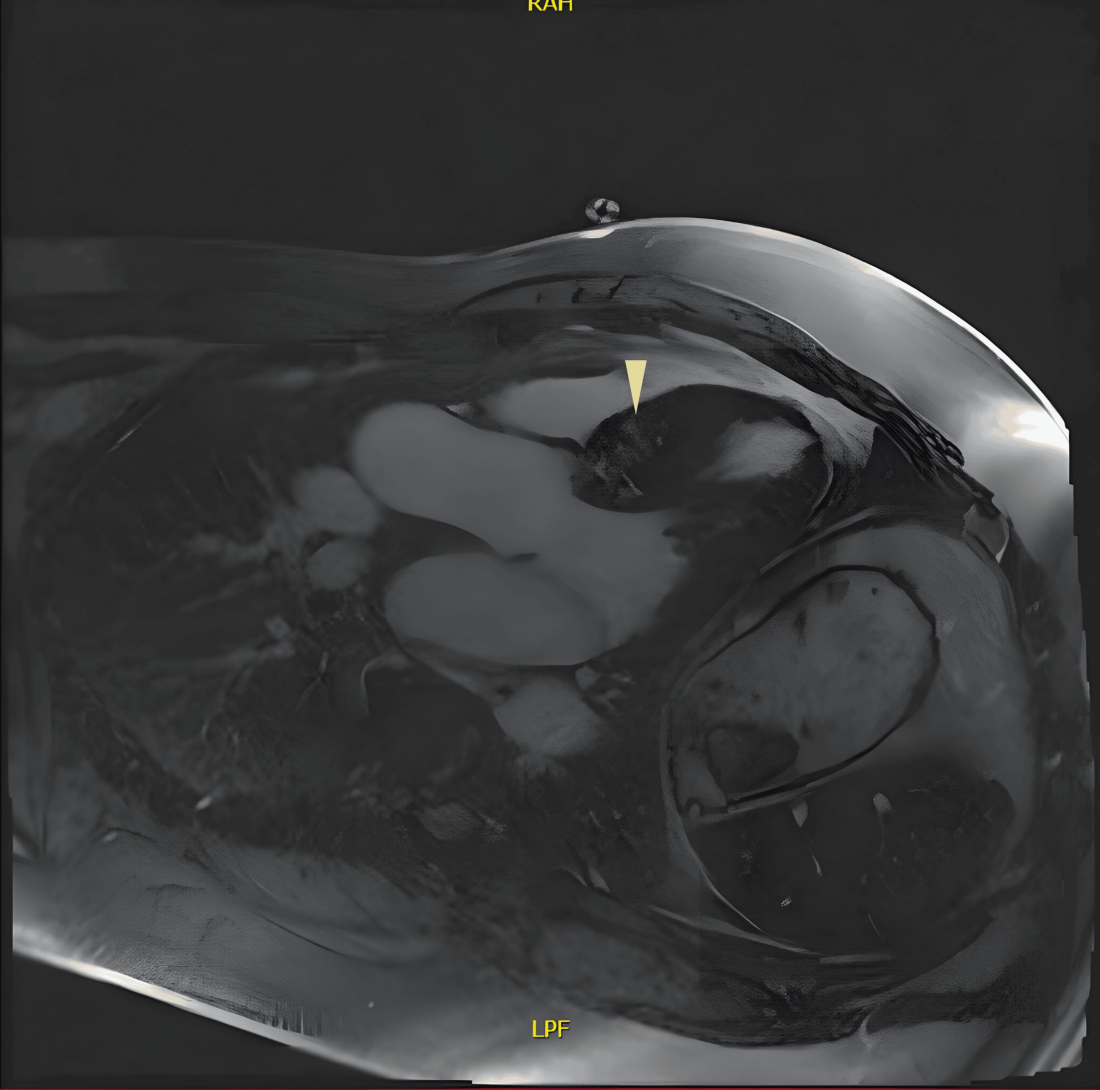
Cardiac magnetic resonance imaging - three-chamber view Extensive mid-wall late gadolinium enhancement (yellow arrow) involving basal to mid anteroseptum.
